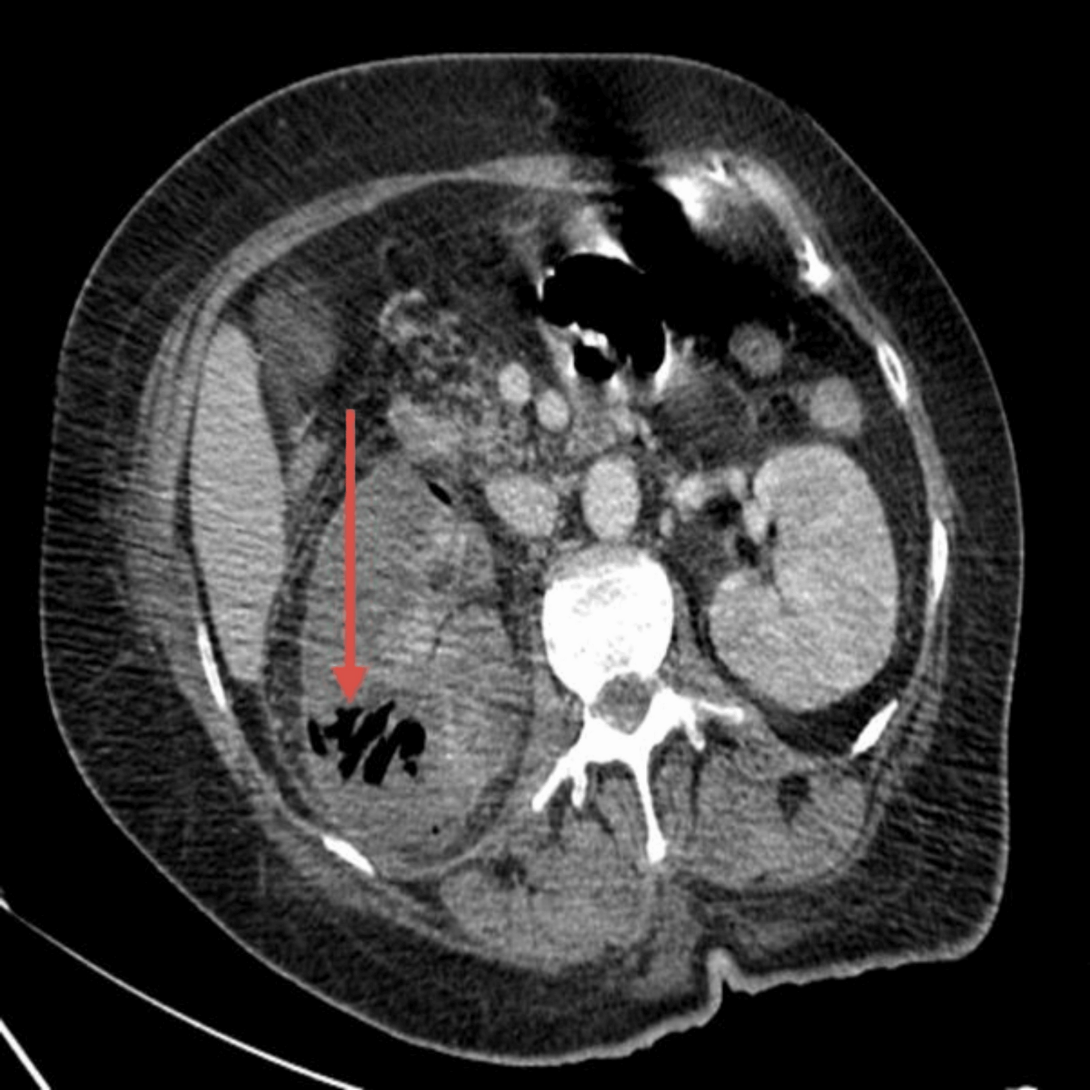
Axial CT scan showing gas within the right renal parenchyma.
